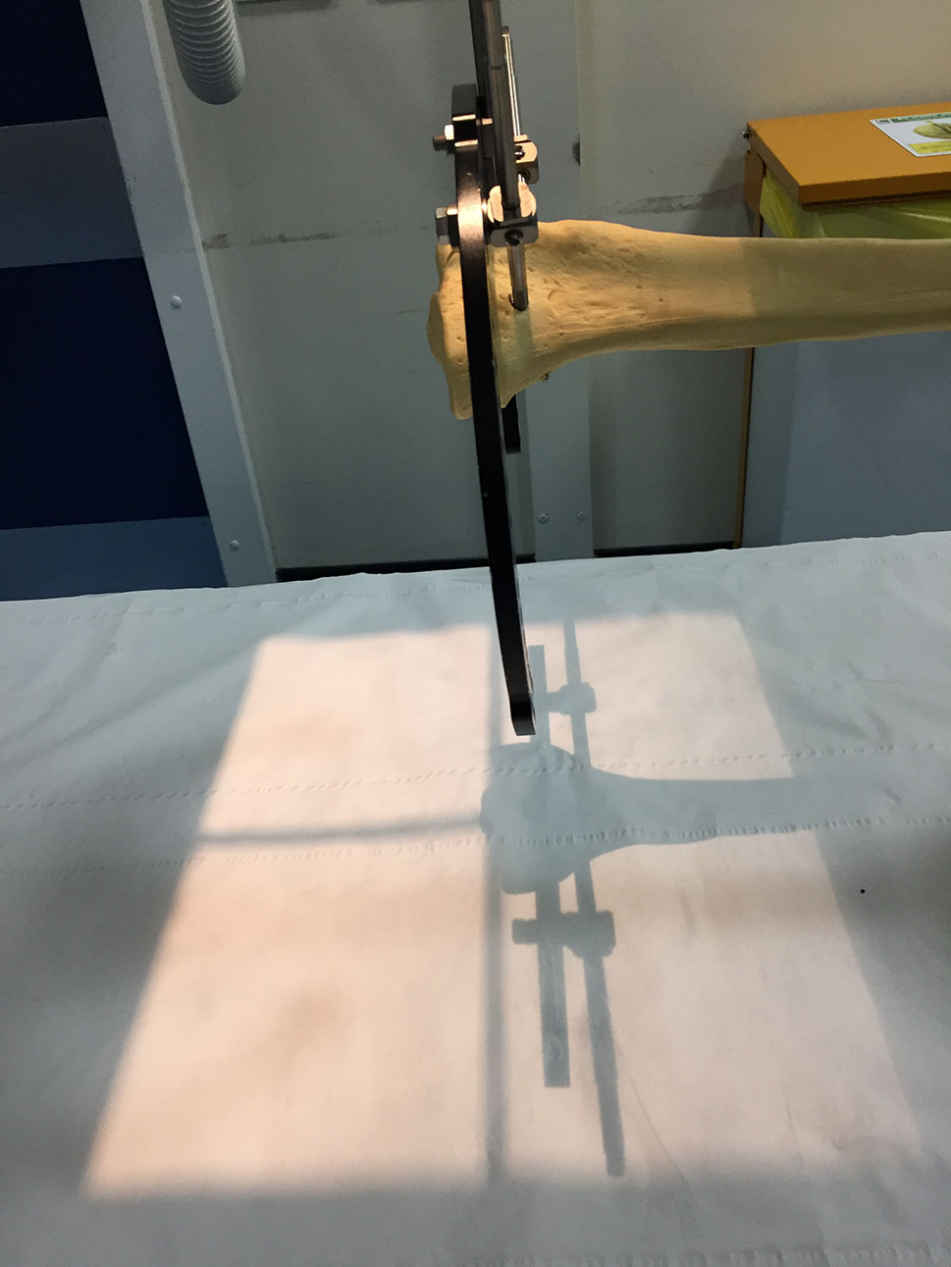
The *shadow* created by the ring is now a *single line* indicating the X-ray beam is orthogonal to the plane of the ring
